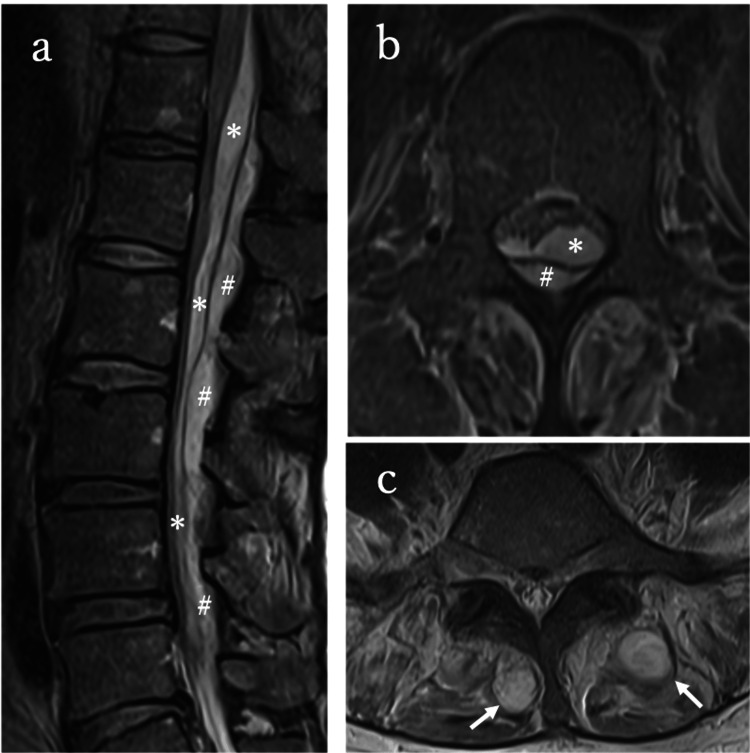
Pre-treatment MRI (a) Sagittal T2-weighted image showing subdural (*) and epidural (#) empyema. (b) Axial T2-weighted image showing subdural and epidural empyema. (c) Axial T2-weighted imaging showing a multifidus muscle abscess MRI: magnetic resonance imaging
